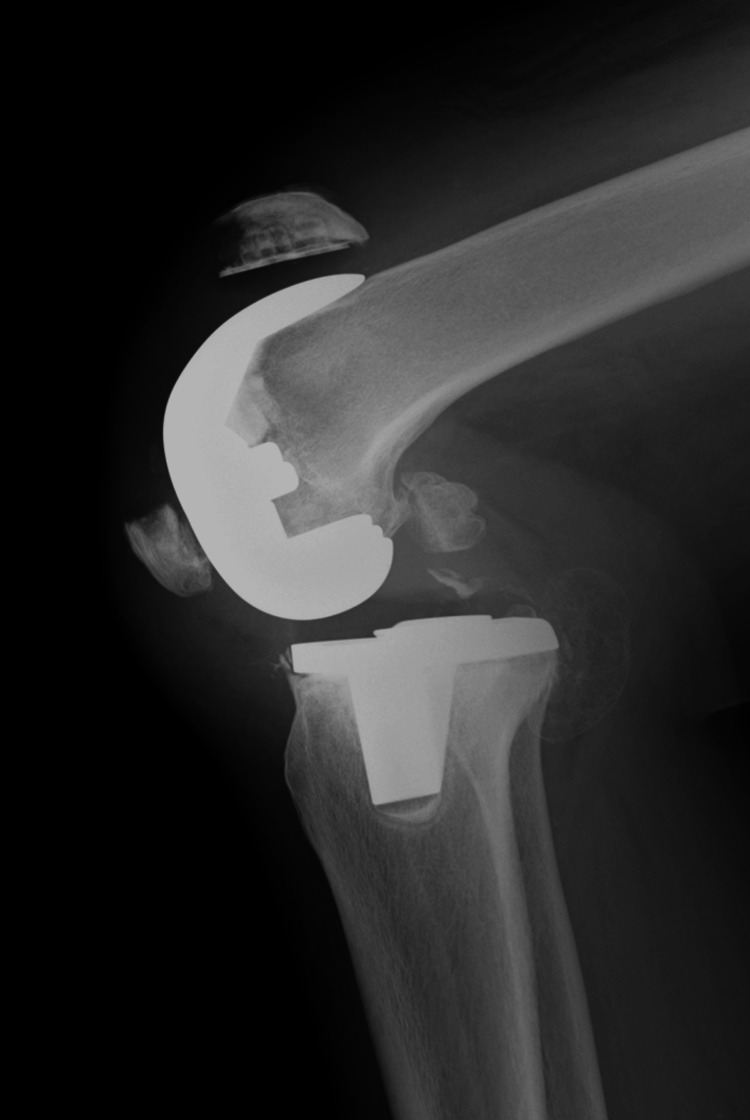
Injury and before initial surgery X-ray imaging at the time of injury revealed a right patellar fracture.
